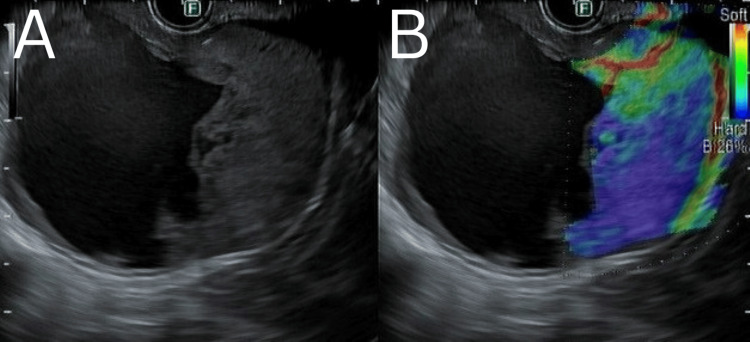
EUS with elastography EUS imaging (A) demonstrates a mixed solid and cystic lesion, with elastography (B) demonstrating an increase in stiffness of the solid component consistent with a mass. Both the cystic component and the solid component were sampled. EUS, endoscopic ultrasound
